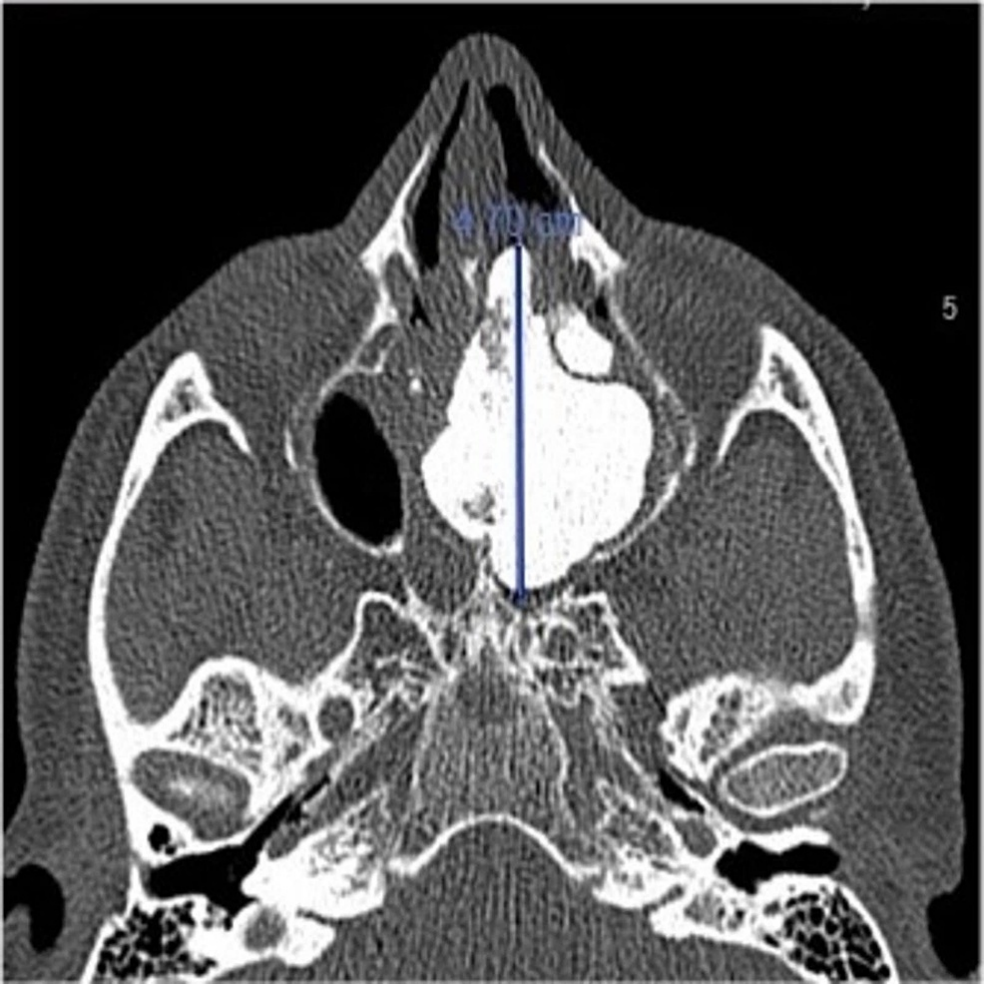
Preoperative CT scan: axial view shows 4.7 x 3.5 cm left ethmoid lobulated hyperdense lesion.
